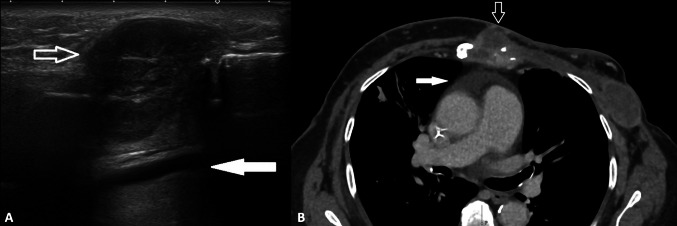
Female patient of 67 years old. B-mode US (A) and CT scans (B). Heterogenous and hypoechoic bone metastasis replaces the echogenic cortical line of the sternum in a patient with breast cancer (empty arrow in A). Deeper to the described lesion, anechoic pericardial effusion is observed (full arrow in A). CT confirms both pathological findings: bone metastasis (empty arrow in B) and pericardial effusion (full arrow in B)
